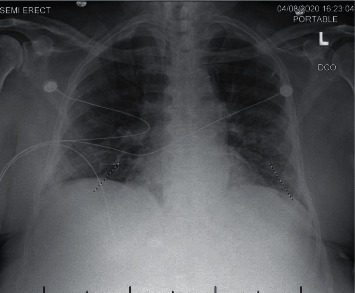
Portable chest X-ray showing bilateral interstitial infiltrates on admission.
